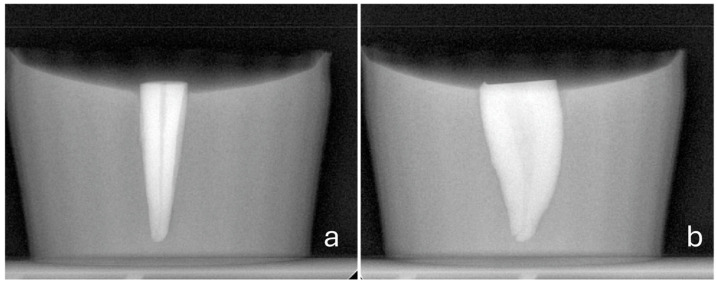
(a) Buccolingual (BL) and (b) mesiodistal (MD) radiographs confirming the vertical positioning of the roots in the acrylic blocks.
